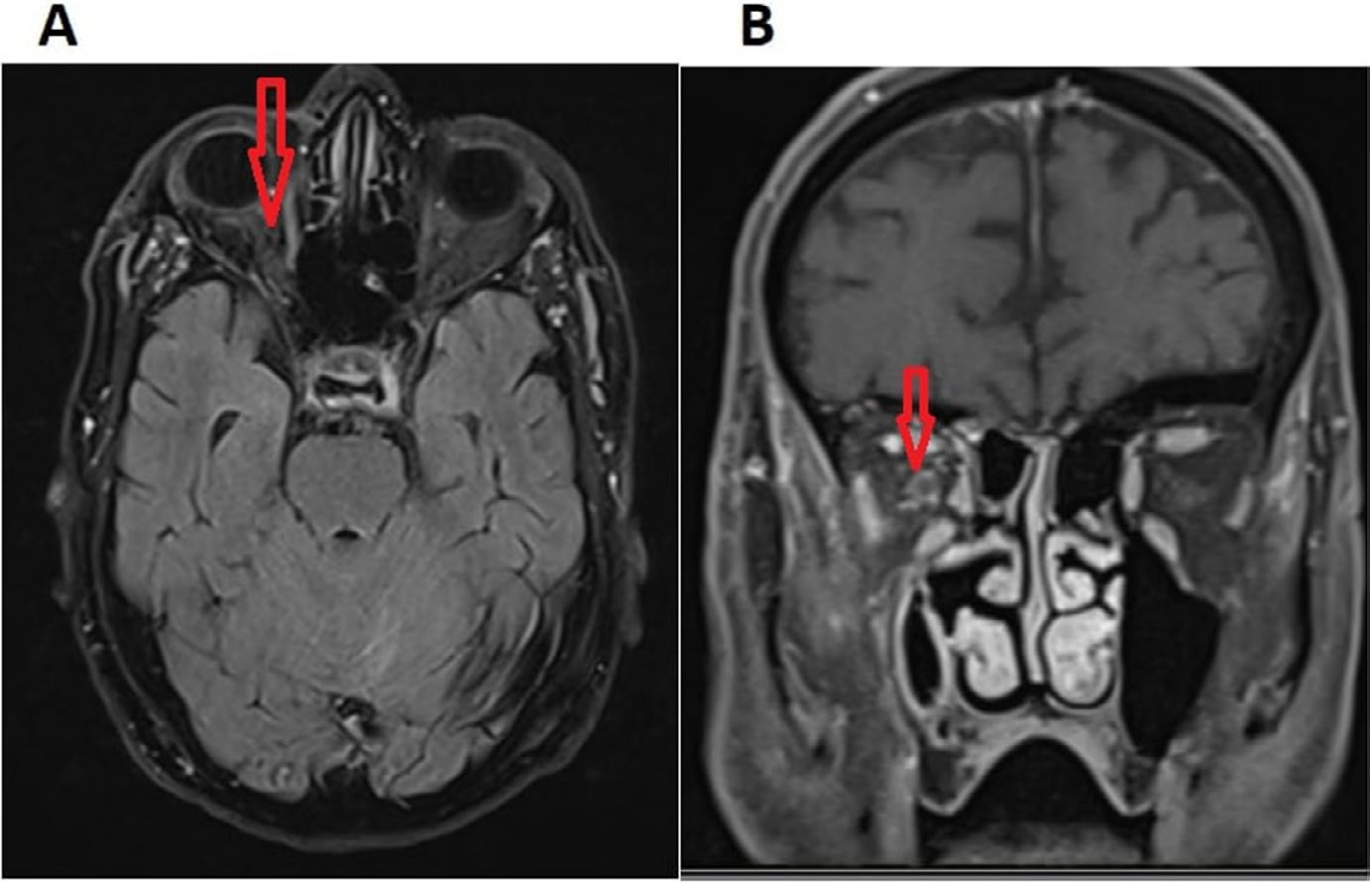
Axial (A) and coronal (B) T1 fat‐suppressed MRI scans with gadolinium enhancement show the orbital apex infiltration with the optic nerve sheath involvement of the right side (red arrow).
